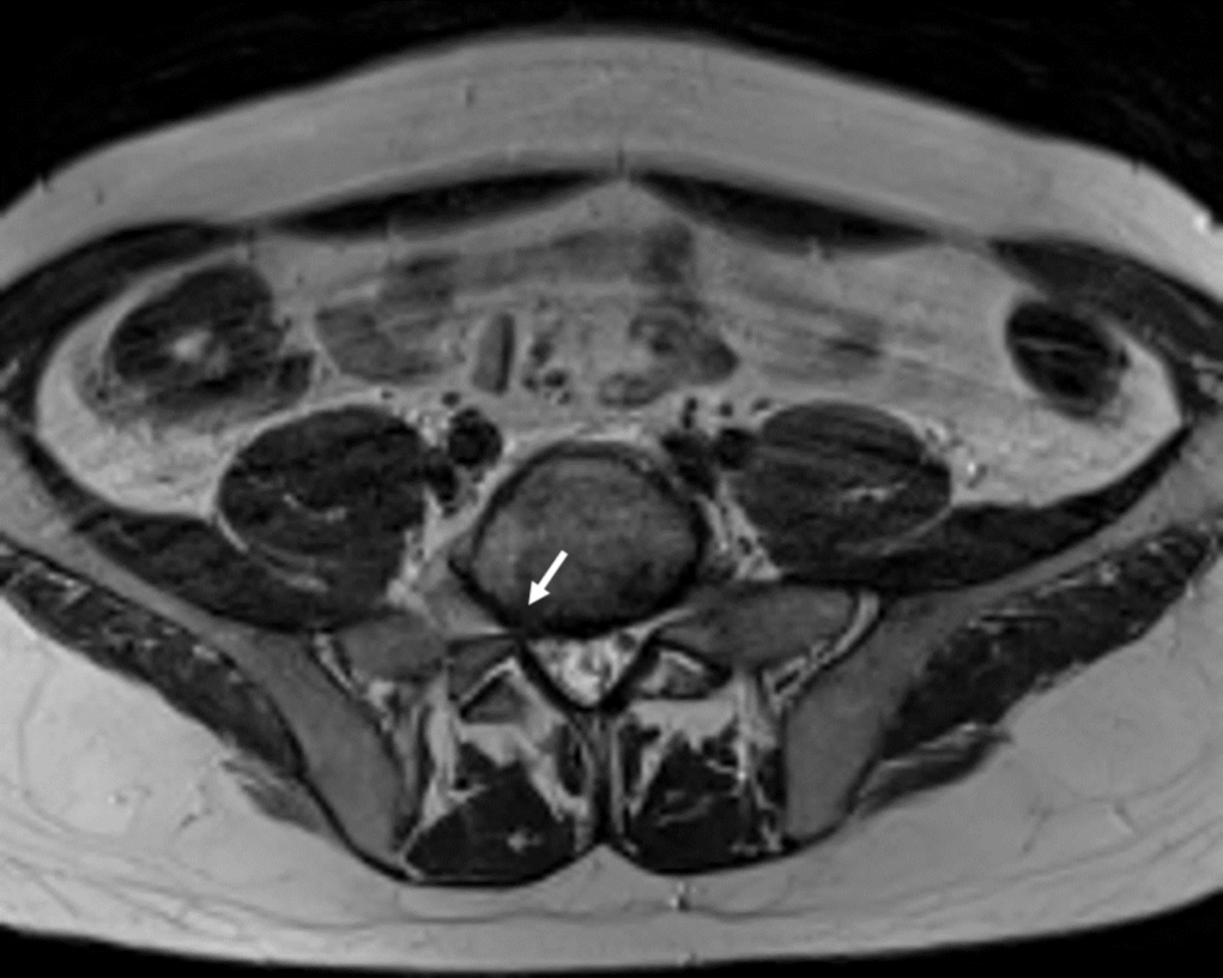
L5/S1 disc level Axial T2 weighted magnetic resonance imaging (MRI) showing L5-S1 disc bulging without significant mass effect (white arrow)
